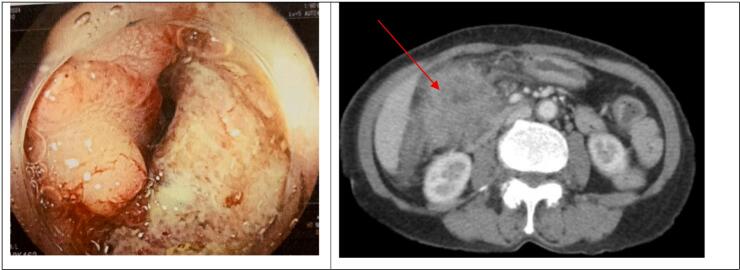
a. An occluding tumor in the hepatic flexure colon in the colonoscopy. b: A tumor 52 × 74 mm in size invaded the pancreatic head and D2 duodenum in the CT scan (red arrow).
